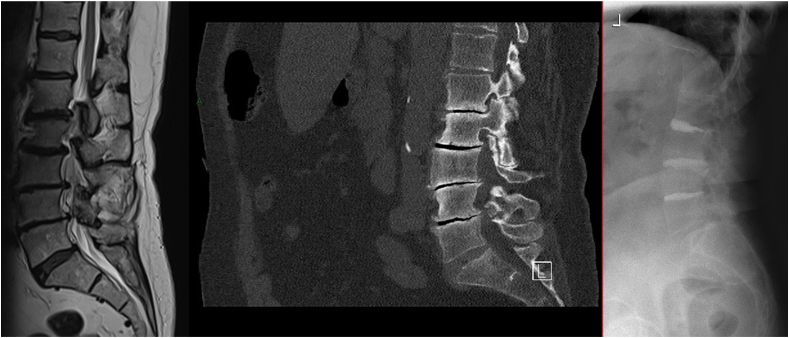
72 year old woman with severe arthritic back pain, bilateral (L5) neurogenic leg pain, hamstring fatigue, poor mobility, high BMI and diabetes. After initial rehabilitation and attempted weight loss, she was treated with L34 & L45 decompression and cement discoplasty (CD) at L23, L34 & L45. No peri-operative or early complications. A: Pre-operative mid-sagittal MRI image displaying multilevel disc degeneration & stenosis. B: Mid-sagittal CT image indicating large abdominal girth and multilevel intradiscal vacuum phenomenon (IDVP) and sclerotic endplates. C: Follow-up post-operative radiograph with preservation of lumbar lordosis. VAS improved from 8/10 (constant) to 4/10 (episodic) back pain and 0/10 leg pain, and Oswestry Disability Score (ODI) improved from 76% pre-operatively to 36% after two years.
